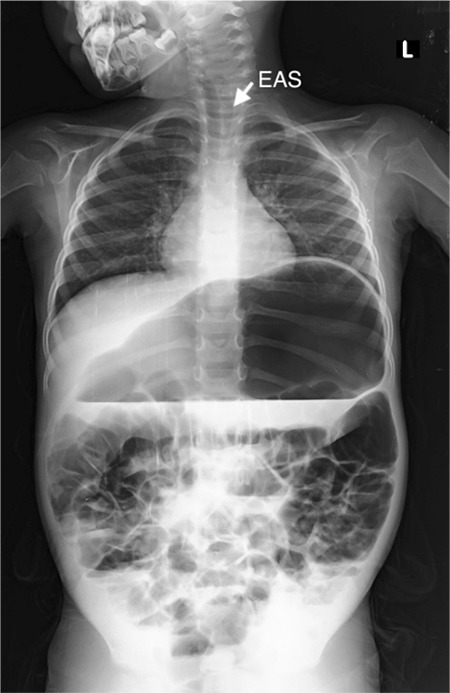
Plain abdominal X-ray shows esophageal air sign and distended stomach, indicative of aerophagia. EAS: esophageal air sign
